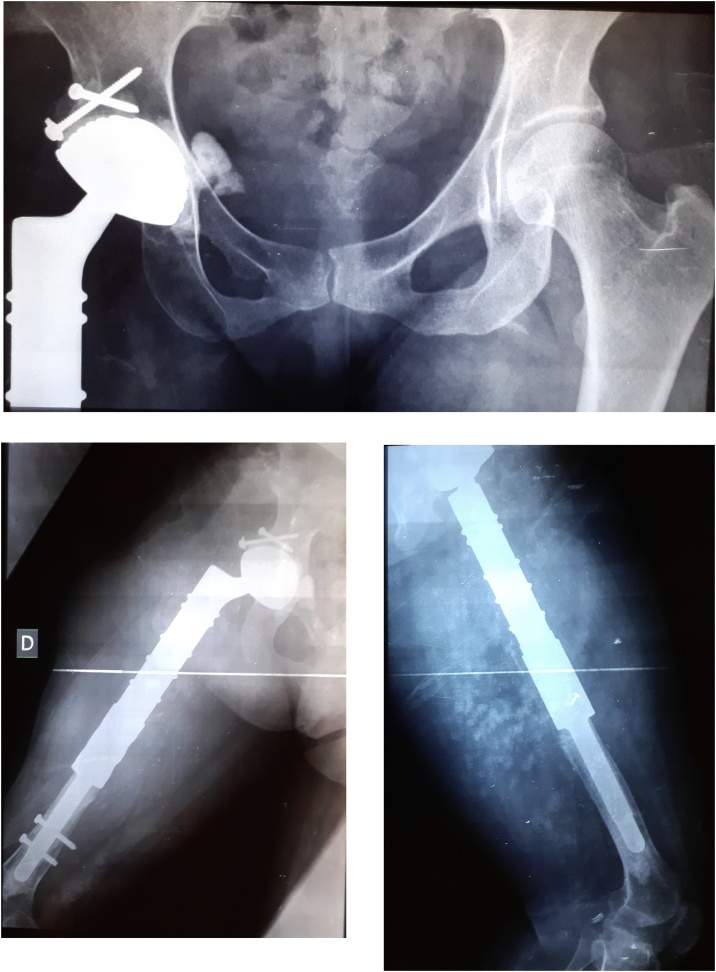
Three years postoperative X-ray images.
